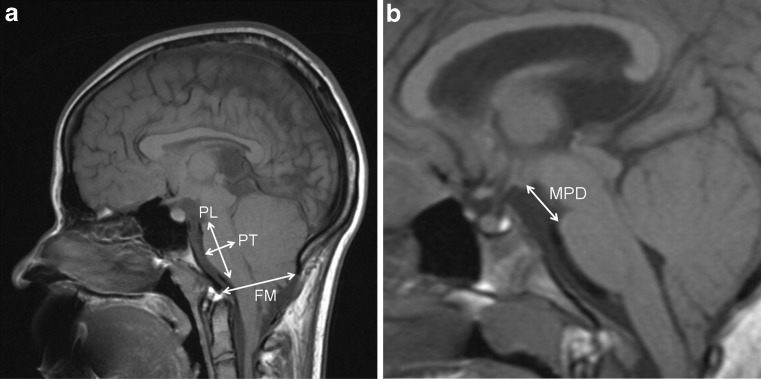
a Sagittal T1-weighted brain MR image of a 16-year-old child with open spinal dysraphism and Chiari II malformation. The *arrows* indicate foramen magnum diameter (*FM*), pons length (*PL*), and pons thickness (*PT*); b sagittal T1-weighted brain MR image of a 8-year-old child with open spinal dysraphism and Chiari II malformation. The arrow indicates mamillopontine distance (*MPD*)
